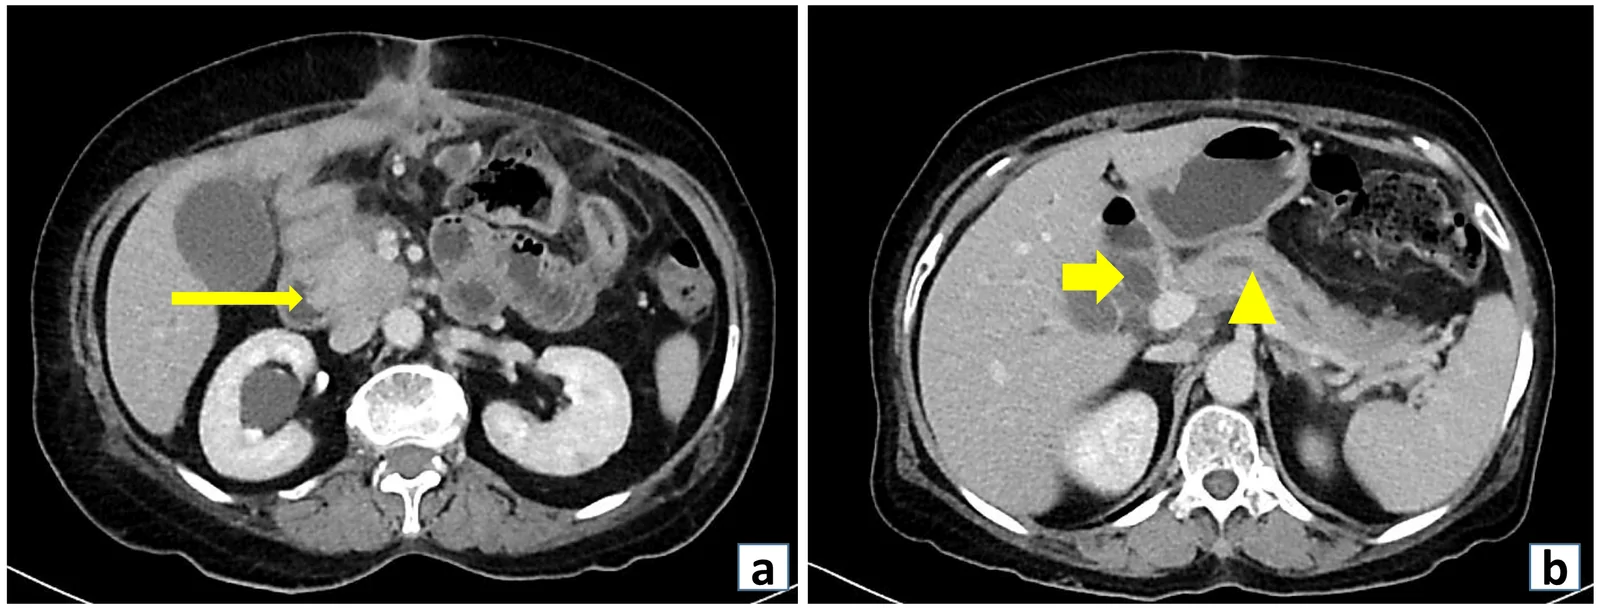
Computed tomography scan of the abdomen with (a) slender arrow pointing to a prominent ampulla and (b) arrowhead pointing to a dilated main pancreatic duct and bold arrow pointing to a dilated common bile duct
